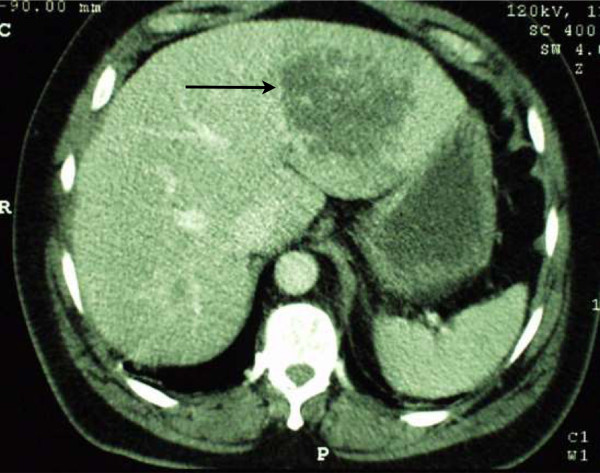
Liver abscess: aspect on admission to hospital. Transverse abdominal CT image of the liver revealing a hypodense area in the left lobe of the liver, measuring 85 mm in width, representing a solid liver abscess.
